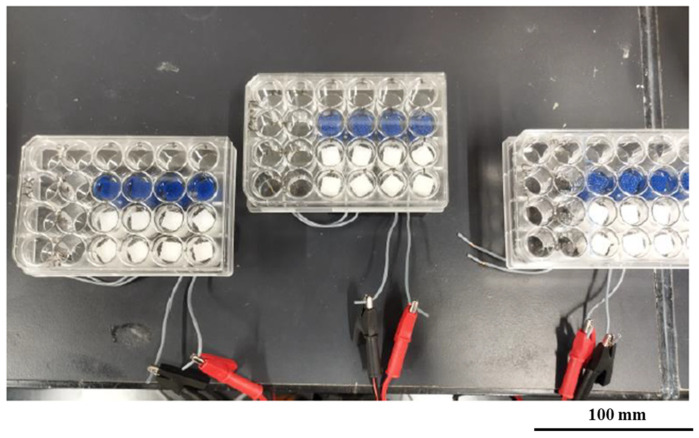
Ultrasound was applied to scaffolds by placing an ultrasound source under 24-well plates.
